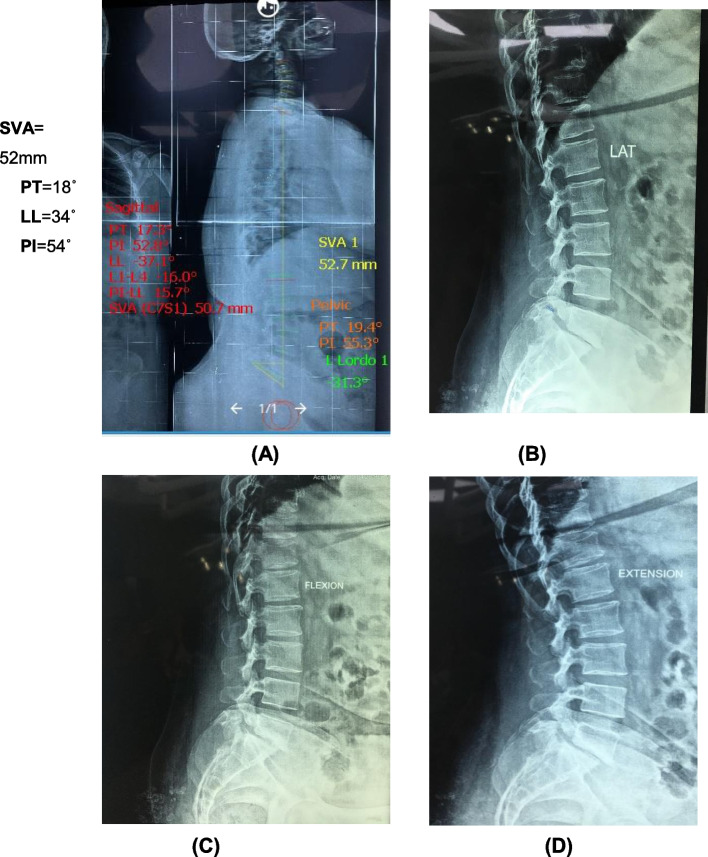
Preoperative X-rays (A) shows the calculation of SVA and other pelvic parameters. B Preoperative lateral view isthmic spondylolisthesis. C, D Flexion and extension views
